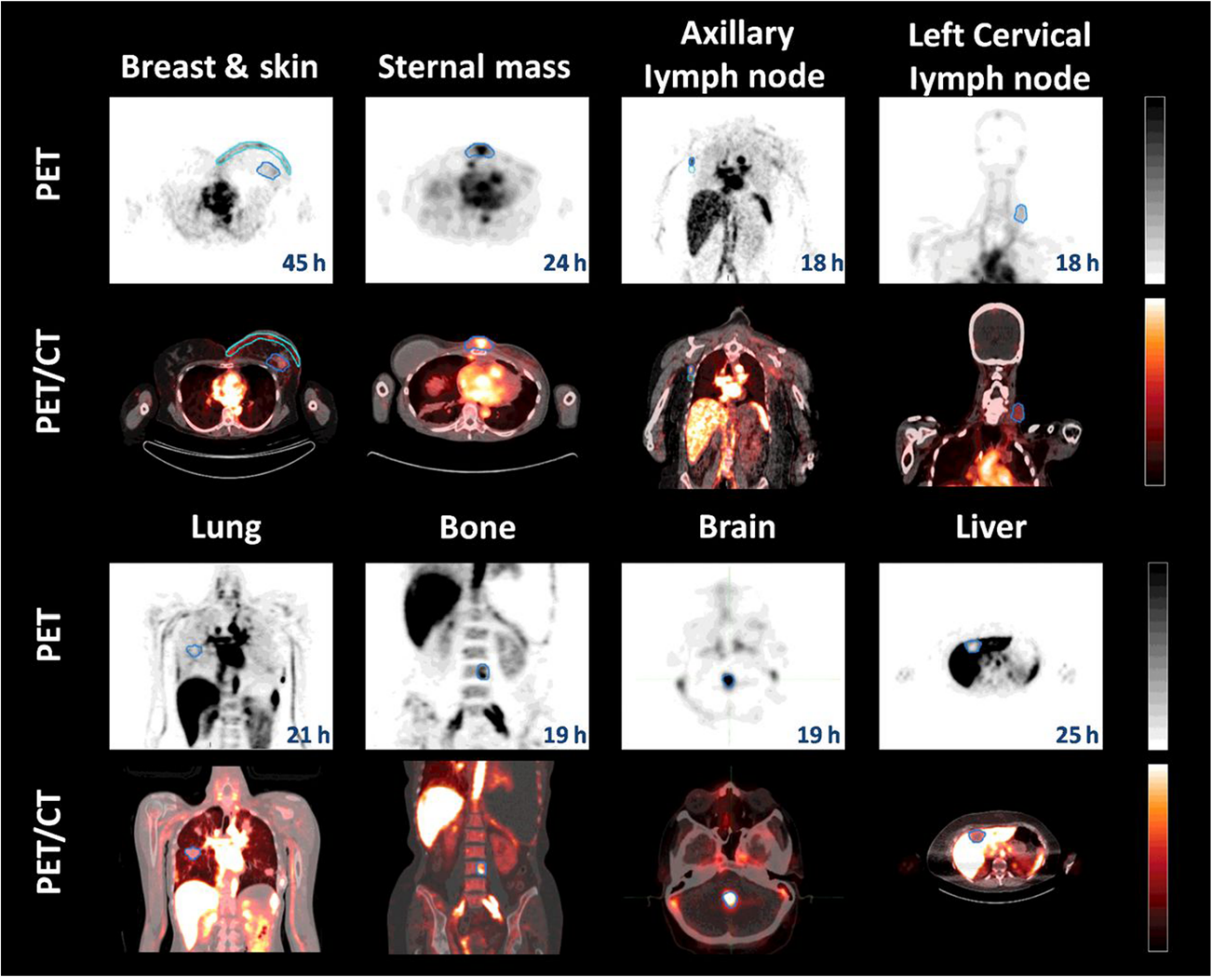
Representative PET and fused PET/CT images of [^64^Cu]-MM-302 in lesions at different anatomic locations. The regions of interest used to measure tumor deposition of [^64^Cu]-MM-302 are shown in blue or turquoise outlines. Adapted with permission from the American Association for Cancer Research: Lee *et al.* [28].
